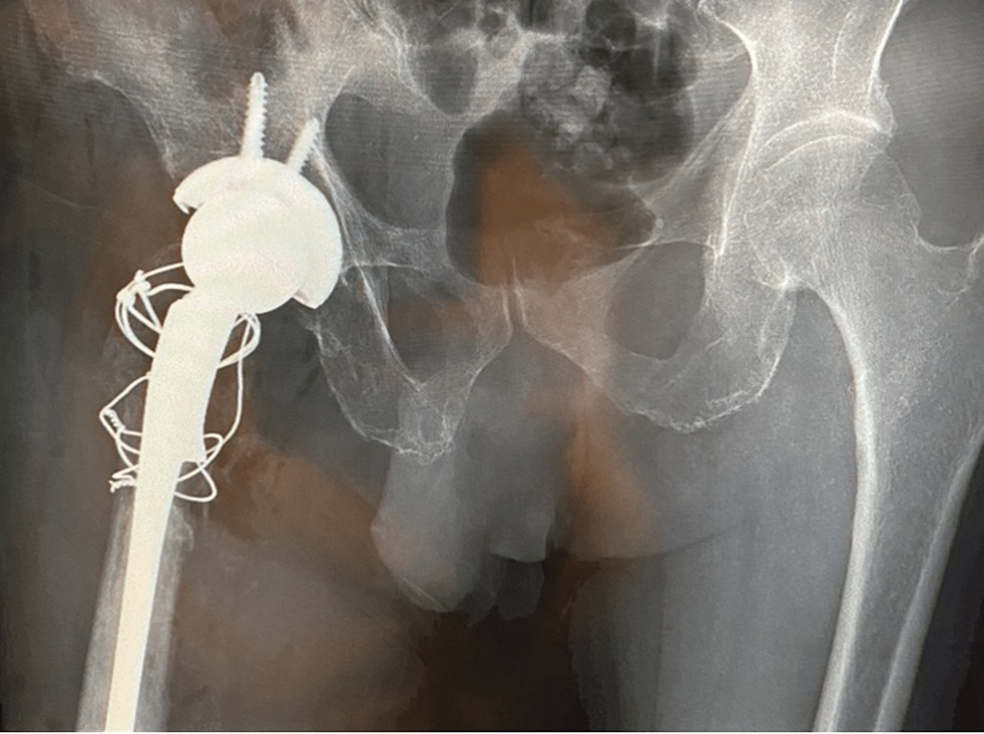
Anteroposterior radiograph of the pelvis showing the reconstructed right total hip arthroplasty.
